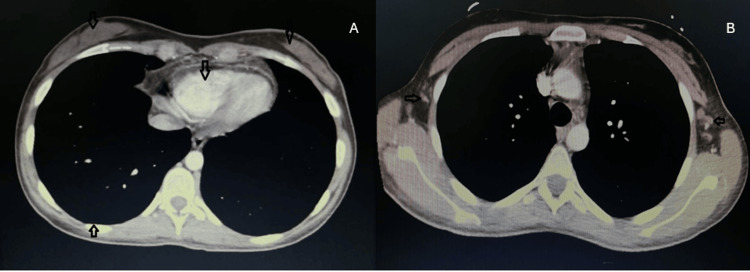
CT scan at three months post treatment showing complete resolution of bilateral breast soft tissue masses and pericardial and pleural effusion and almost complete resolution of mediastinal mass (A) and decrease in the size and number of axillary LN (B). LN: lymph nodes.
